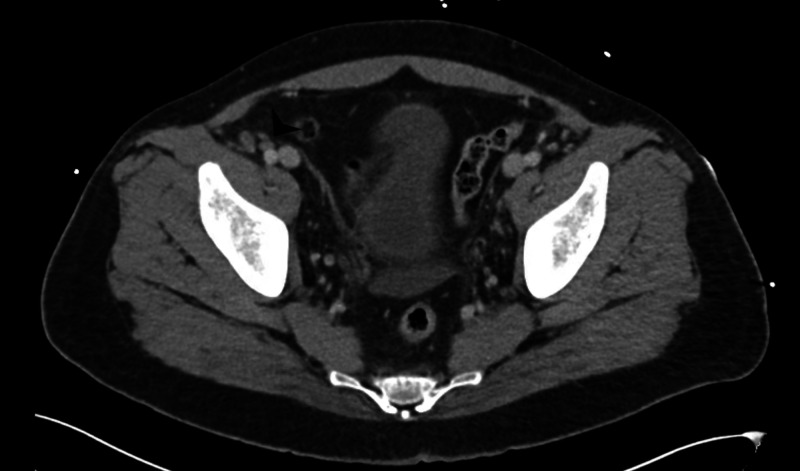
CT abdomen and pelvis with IV contrast demonstrating a 1.3 x 0.8 cm right external iliac lymph node.
